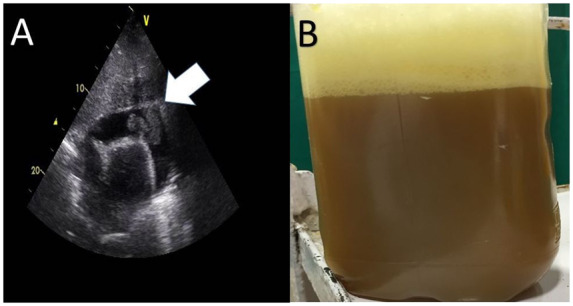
(*A*) A transthoracic echocardiogram four-chamber view from the subxiphoid approach showed massive pus collection in the pericardial cavity leading to cardiac tamponade. (*B*) A 700 mL brown colour pus-like material (Anchovy sauce) was aspirated from the pericardium.
